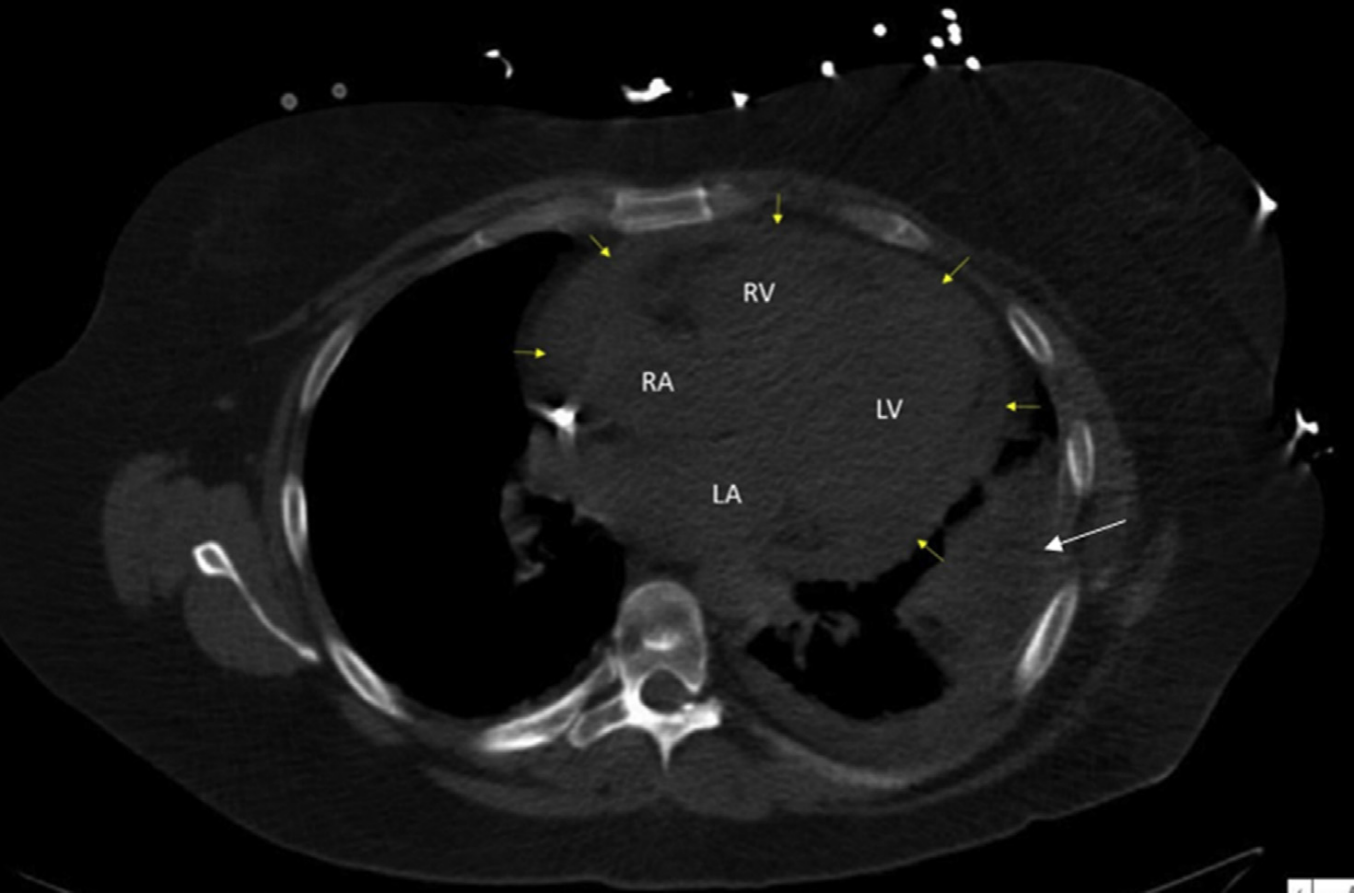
Chest CT scan axial view showing moderate to large circumferential pericardial effusion (*yellow arrows*) and left pelural effusion (*white arrow*). *LA*, Left atrium; *LV* left ventricle; *RA*, right atrium; *RV*, right ventricle.
